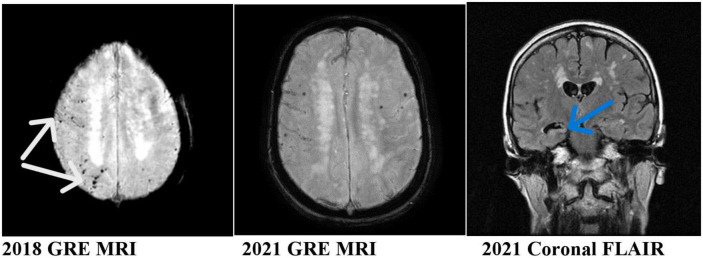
MRI of the patient showing progressive asymmetric (right worse than left) hemisphere amyloid and hippocampal atrophy. White arrows: microhemorrhages, sites of cerebral amyloid angiopathy. Blue arrow: right hippocampal atrophy.
